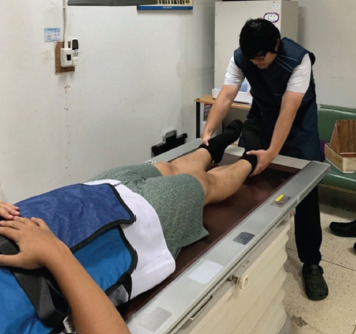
Conventional radiograph positioning by medical personnel.
